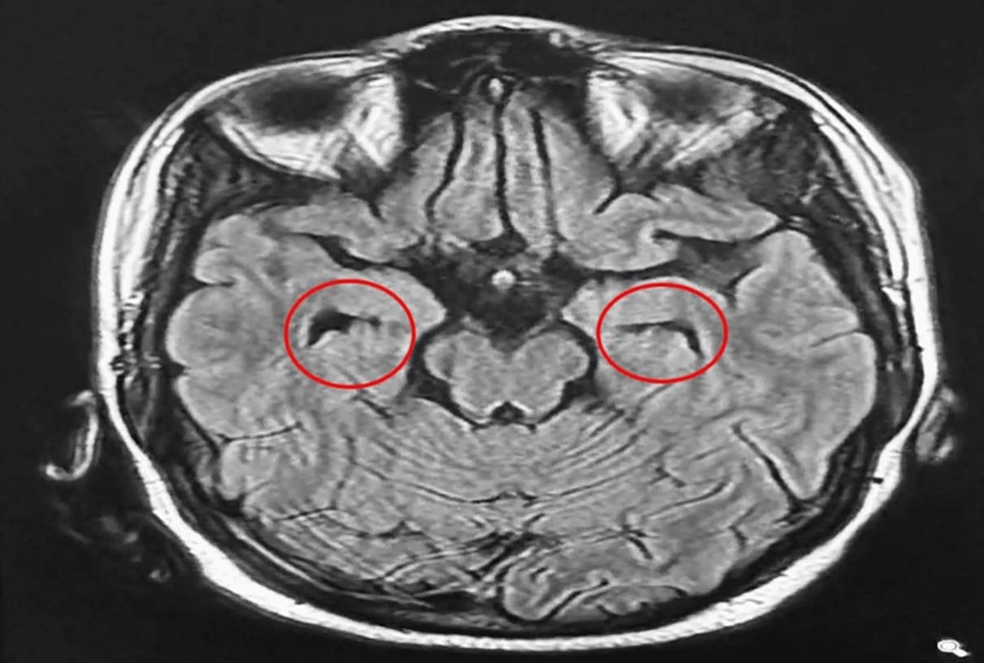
MRI brain without contrast showed mesial temporal lobes atrophic changes MRI: Magnetic Resonance Imaging
